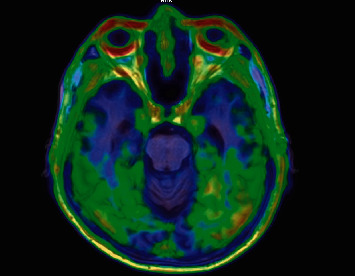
Fusion images between [^18^F]PI-2620 PET and T1-weighted MRI in an AD patient. Intense tau deposition was observed in the bilateral temporal and occipital regions.
